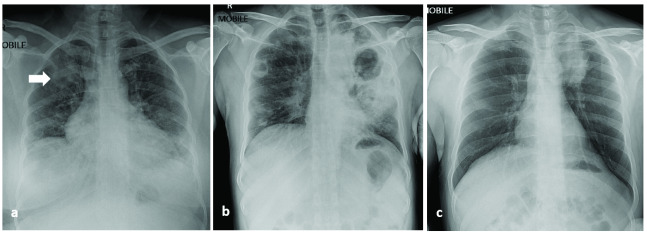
Admission chest X-ray for case 1 (a), case 2 (b) and case 3 (c)
